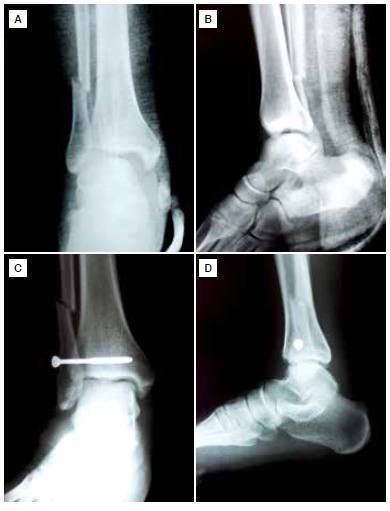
and B) Weber C ankle fracture with tibiofibular diastasis, (C and D). Postoperative radiograph with syndesmosis-only fixation.
